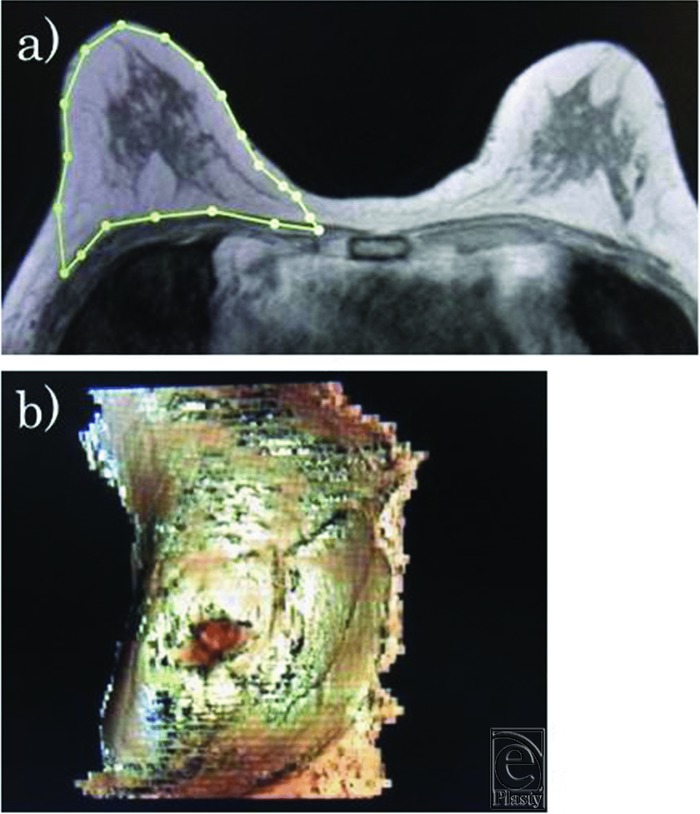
Breast volume measurement by magnetic resonance imaging. (a) Outline of breast tissue. (b) Reconstructed 3-dimensional image and calculation of the breast volume.
